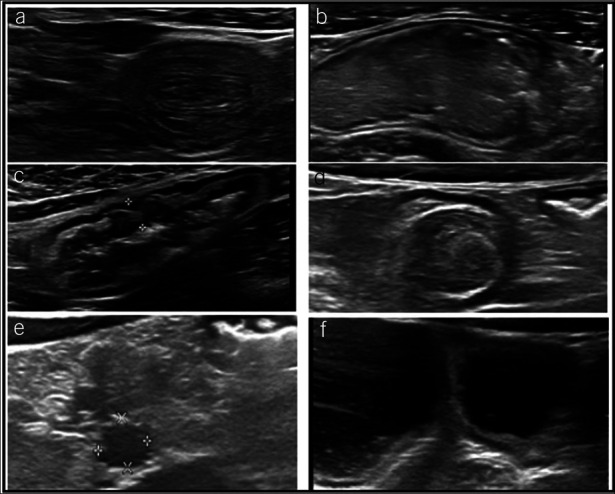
Ultrasonographic findings assessed in 86 patients with CF. (a) Small-bowel intussusception as the sliding part of the intestine into an adjacent part of the intestine. (b) Inspissated intestinal contents. (c) Bowel wall thickened tract (≥3 mm). (d) Thickened appendix biparietal diameter (>6 mm). (e) Enlarged mesenteric lymph nodes in a hypertrophic mesenteric adipose tissue, defined as a hyperechoic and inhomogeneous area surrounding the thickened bowel wall. (f) Intestinal dilatation (>25 mm). CF, cystic fibrosis.
